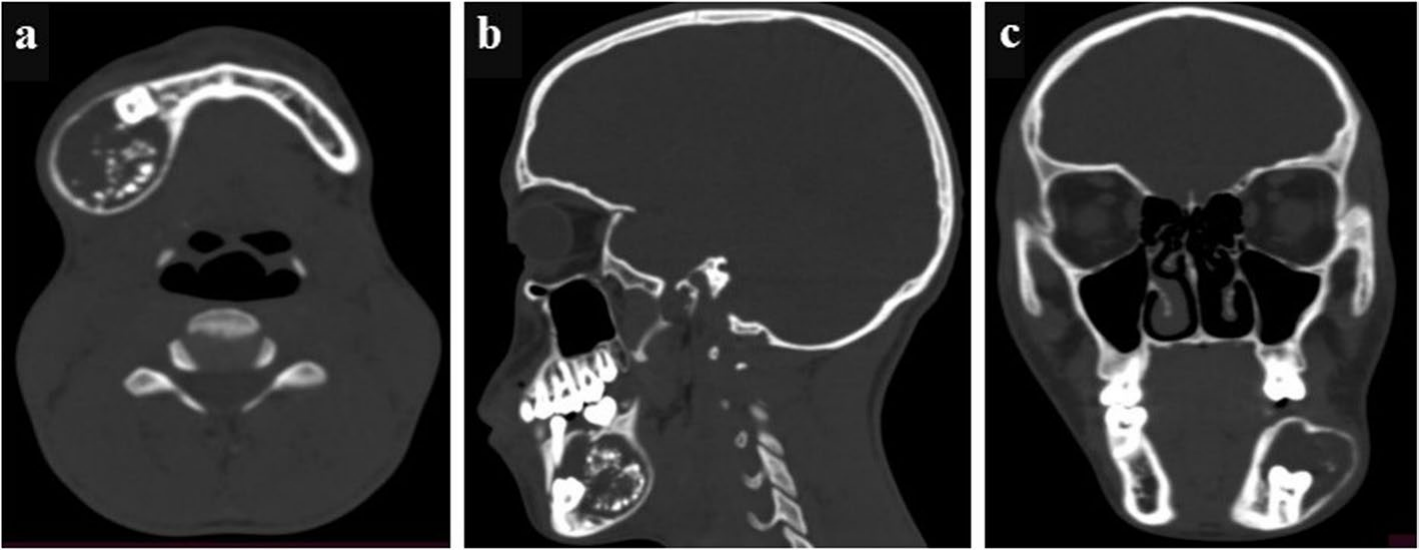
Radiographic features of the tumor. CT scan with contrast of the patient with (a) axial, b sagittal and (c) coronal views respectively demonstrate a well-defined unilocular radiolucency with multiple radio-opaque foci in the right mandible having a dentigerous type of association with the crown of a developing third molar; tooth displacement & root resorption w.r.t permanent second molar is seen. The buccal and lingual cortices are intact but show expansion
